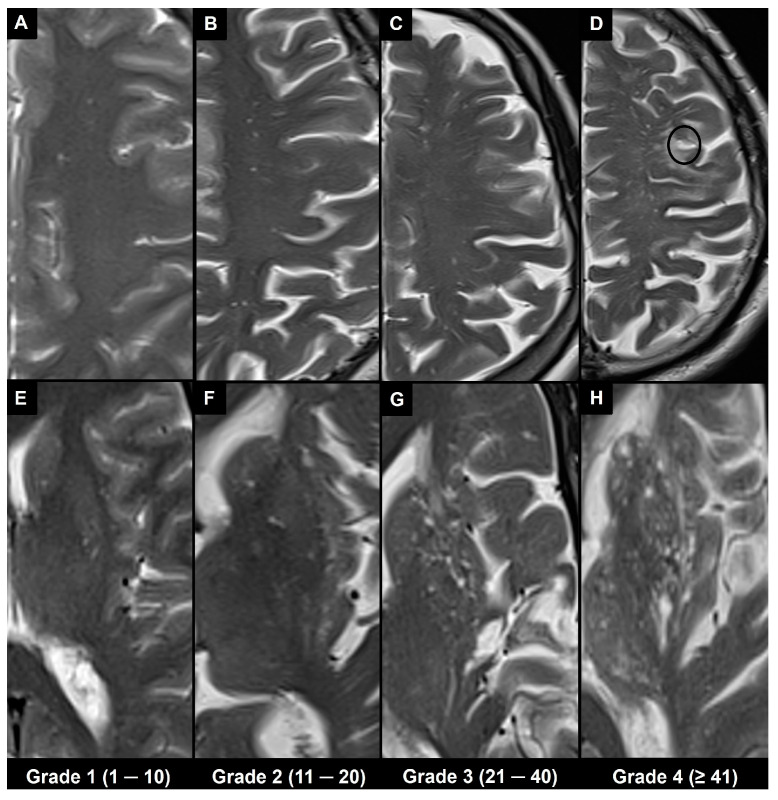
The 1.5 T magnetic resonance imaging T2 turbo spin echo images in different patients show the Potter scoring system based on counting visible PVS. Each hemisphere is scored separately for PVS, and the higher score of the two is used, as indicated at the bottom. (A–D) Axial slices at centrum semiovale level (type II). (E–H) Axial slices at basal ganglia level (type I), where H corresponds to the “état criblé” or “status cribrosum” described by Durand-Fardel in the basal ganglia. Note also the presence of a lacune in (D) (black circle) and that PVS in the centrum semiovale can be observed as they approach the cortex; their dilation increases as they reach the inner edge of the cortex; however, they cannot be seen as they pass through the cortex.
